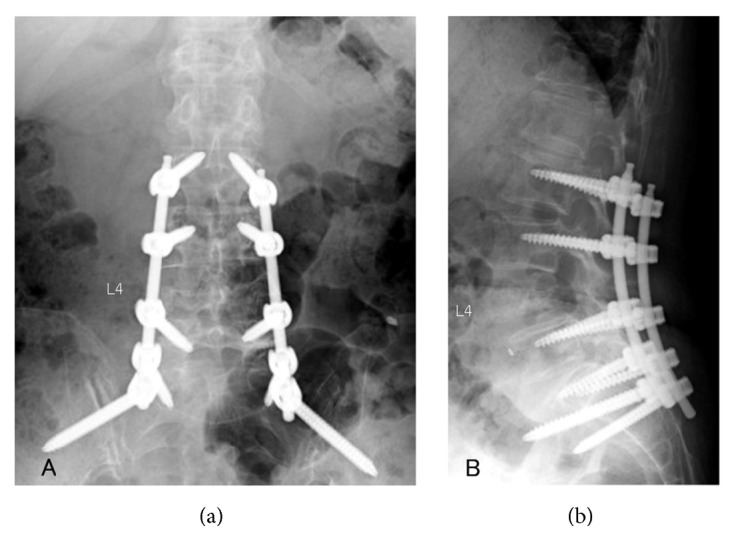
Immediately after minimally invasive spine stabilization without decompression (L2-S2AI) and biopsy of the L4 lumbar vertebrae through the right pedicle were performed. (a) Posteroanterior view on radiography. (b) Lateral view on radiography.
